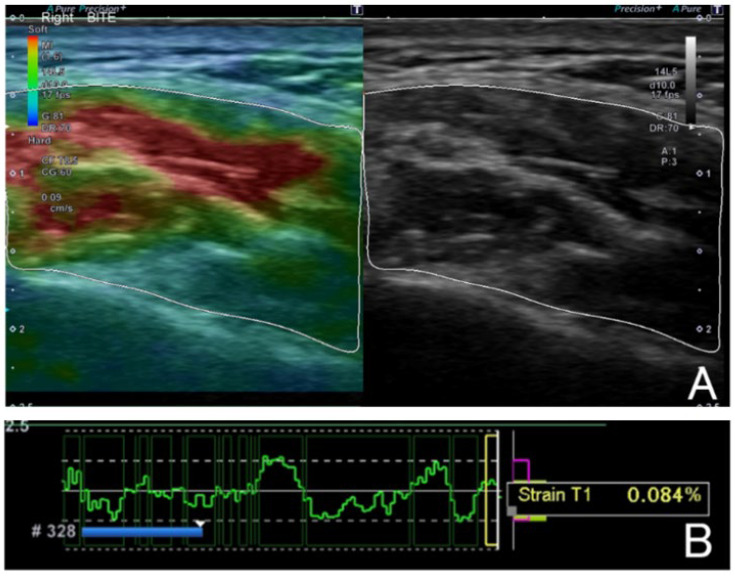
Ultrasound elastography image. Regions of interest (ROI) are indicated by white rectangles and were set to masseter muscle excluding fascia and fat. Color coded from red (high revel strain ratio) to blue (low level strain ratio) according to the hardness of tissue by B-mode images (A). There is a scale that shows the rate of compression and relaxation cycles, and the masseter muscle area is selected when it is constant (B).
